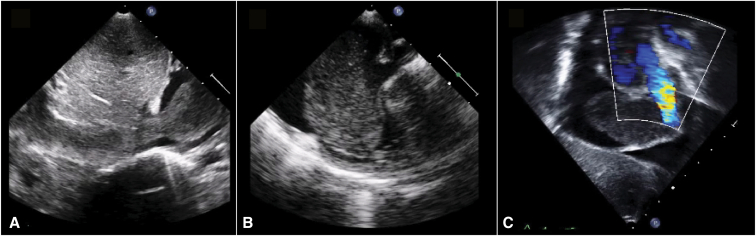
Preoperative (A) subcostal and (B and C) transthoracic echocardiography of patient 3 showing the intracardiac component of the tumor thrombus.
